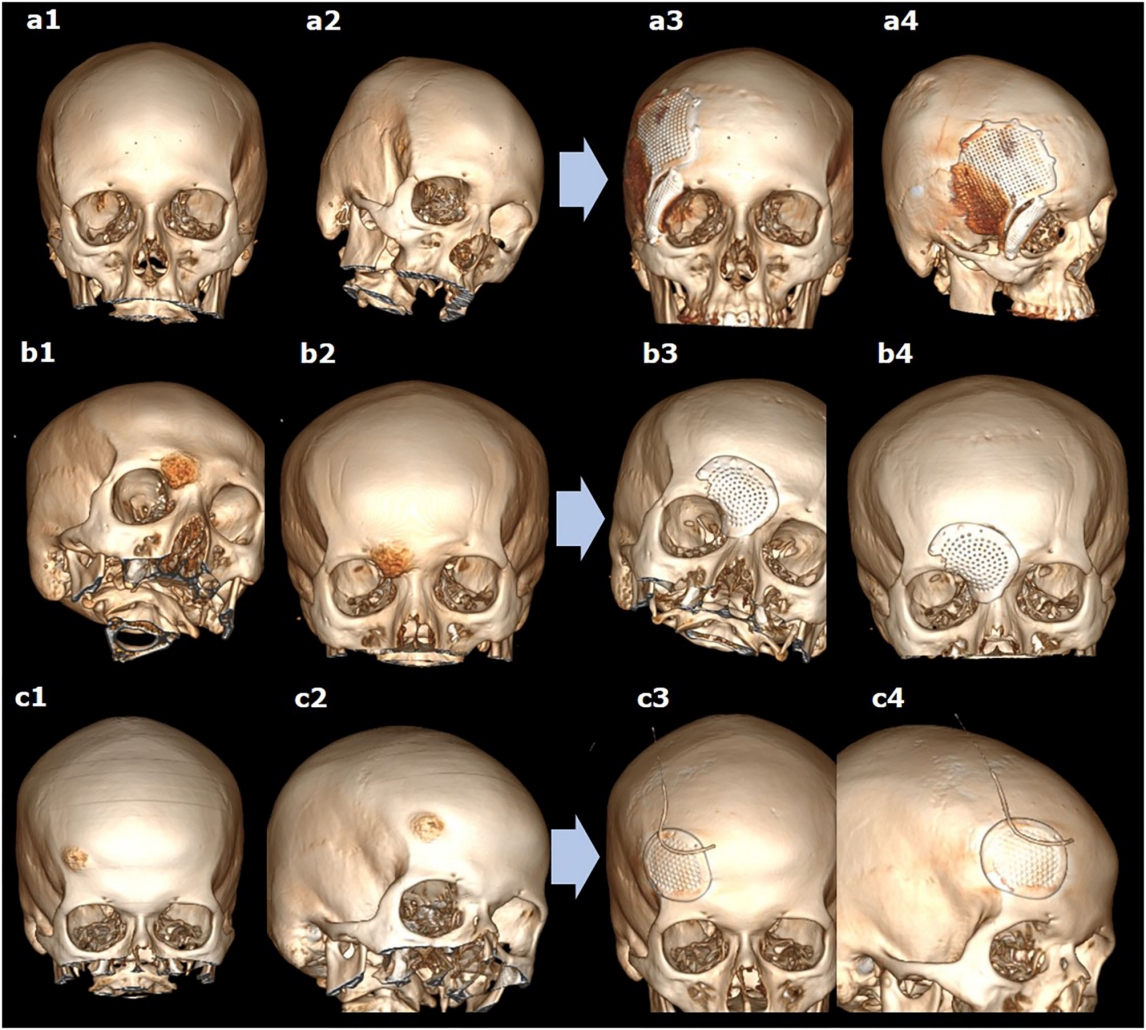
The 3D reconstructions of CT scans before (a1–c1,a2–c2) and after (a3–c3,a4–c4) simultaneous resection of bone tumor and titanium cranioplasty in different cases of lesions in the fronto-orbital region, using two (a1–a4) or one (b1–b4,c1–c4) implants.
